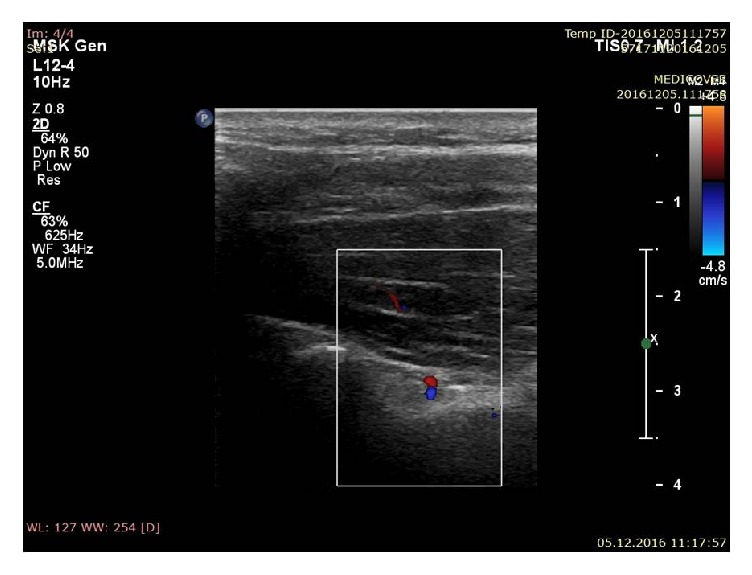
Ultrasonographic investigation of the suprascapular notch region (color Doppler) (stage IV of protocol); blue: suprascapular vein; red: suprascapular artery.
